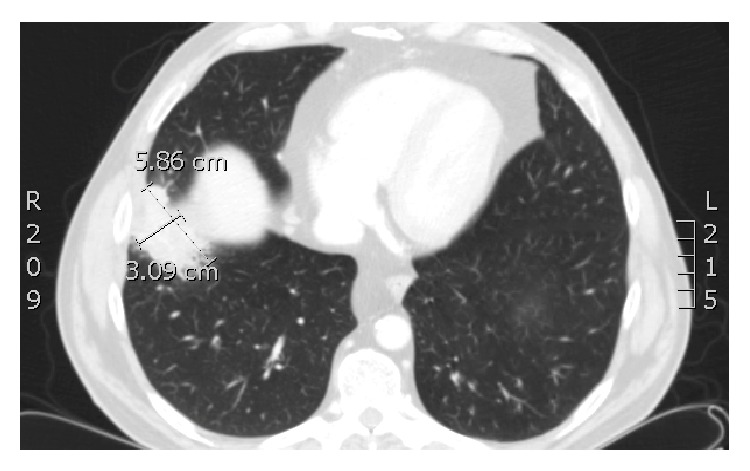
Gallstone-associated subpulmonic fluid collection on preoperative computed tomography measuring 3.09 cm × 5.86 cm.
